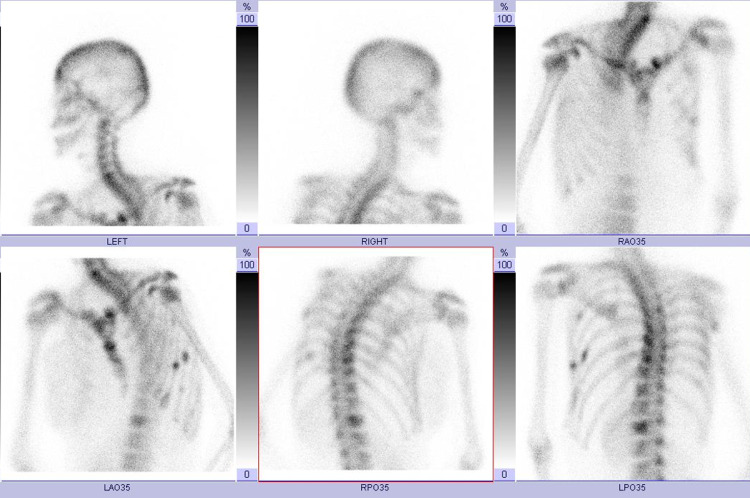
Bone scintigraphy images showing increased tracer uptake in some thoracic spines and ribs.
